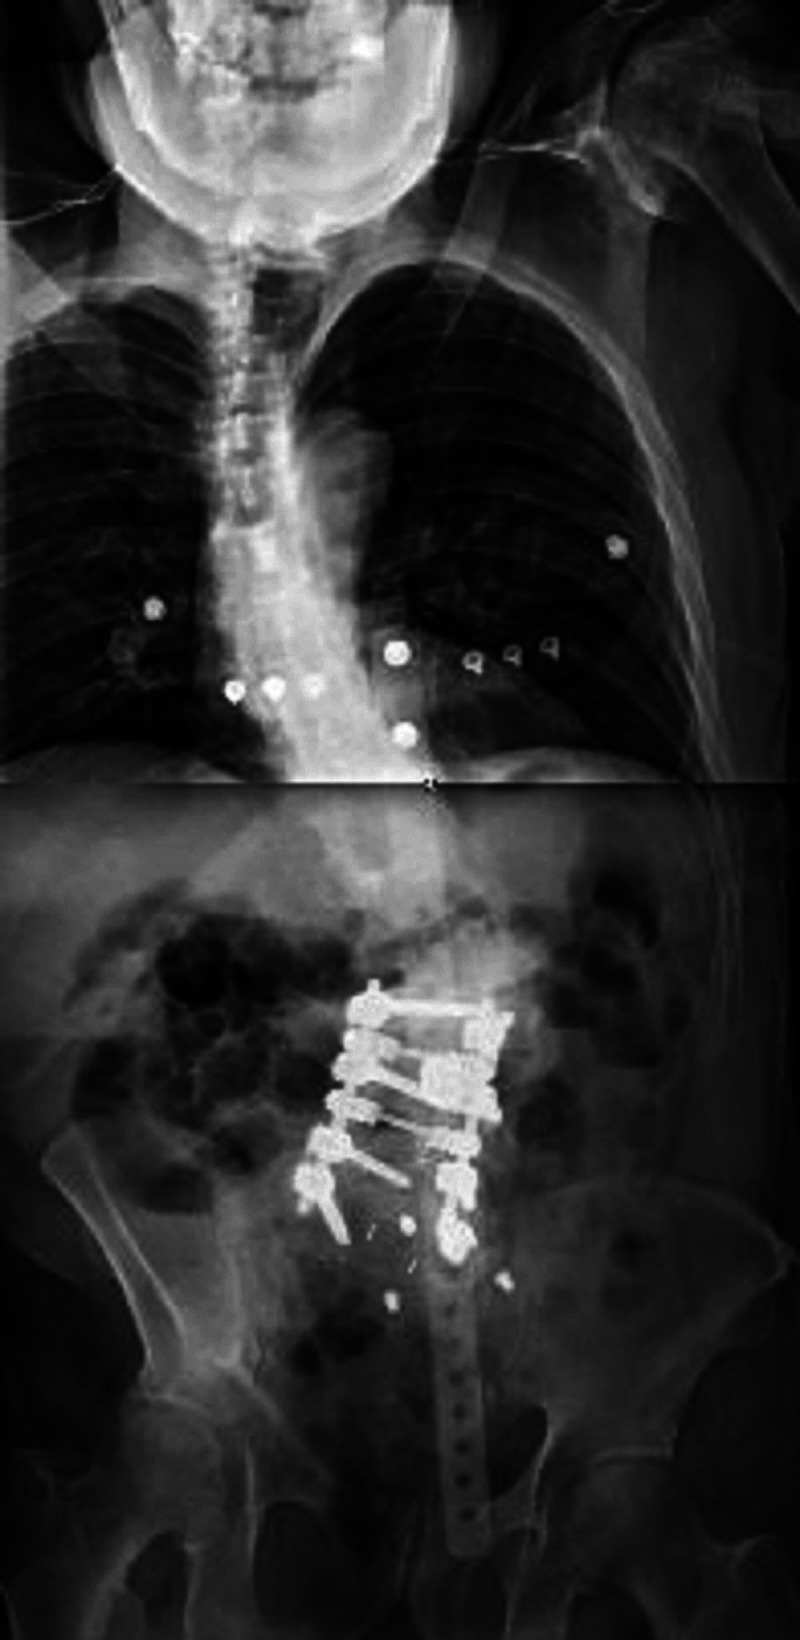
Preoperative standing upright scoliosis x-ray films show the severe S-shaped scoliotic curvature of the patient’s thoracolumbar spine with coronal imbalance prior to kickstand rod surgery.
